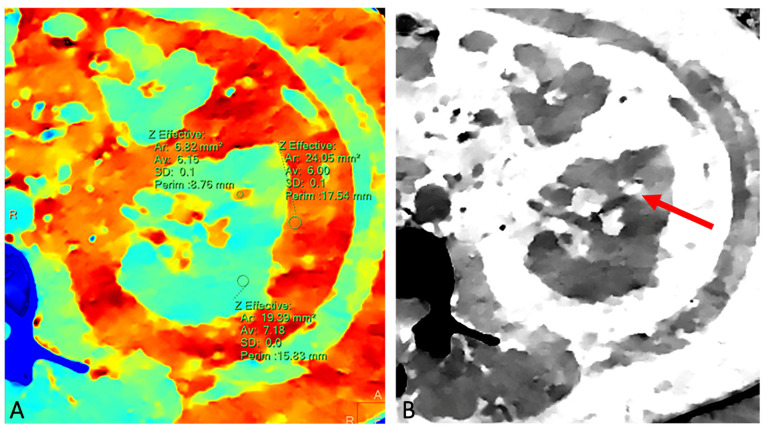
Unenhanced z-effective map CT image demonstrating that the atomic number of small intralesional hypodensity (6.15) is very close to that of perirenal fat (6.0) (A). Unenhanced fat map CT image showing that the attenuation of the small intralesional component (red arrow) increases, as does that of the perirenal and intra-abdominal fat (B).
